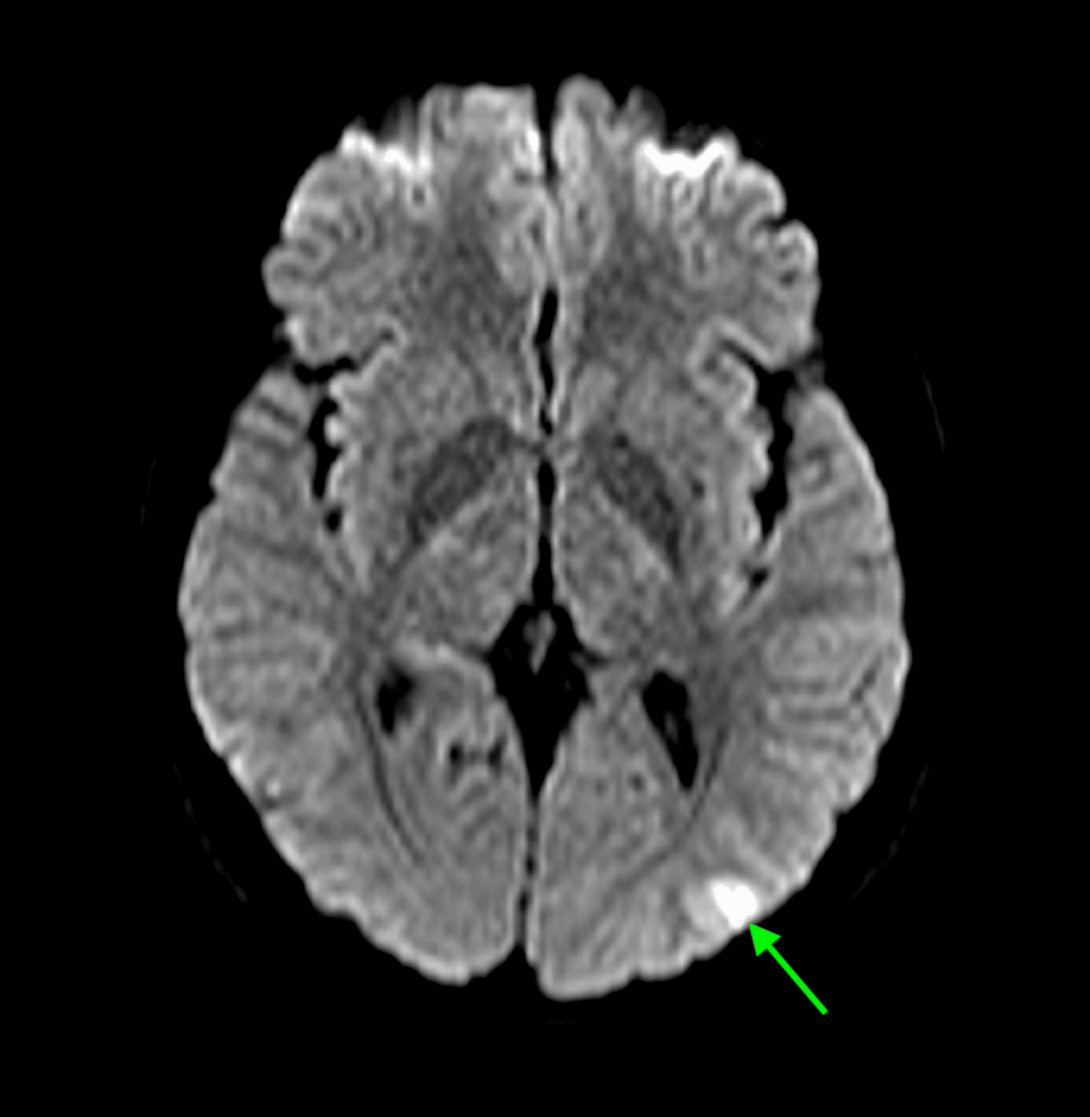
Diffusion weighted MRI of the brain Initial axial diffusion-weighted MRI of the brain demonstrating a hyperintense lesion in the left parieto-occipital cortex (green arrow), initially suggestive of acute infarction or seizure-related changes.
